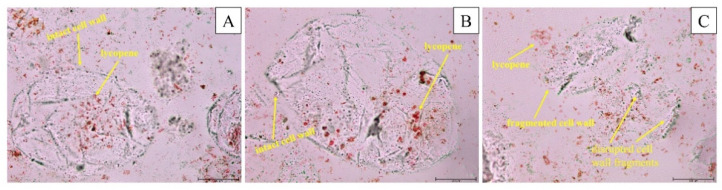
Microstructure of traditional tomato vinegar (A), pasteurized tomato vinegar (B) and ultrasound-treated tomato vinegar (C).
